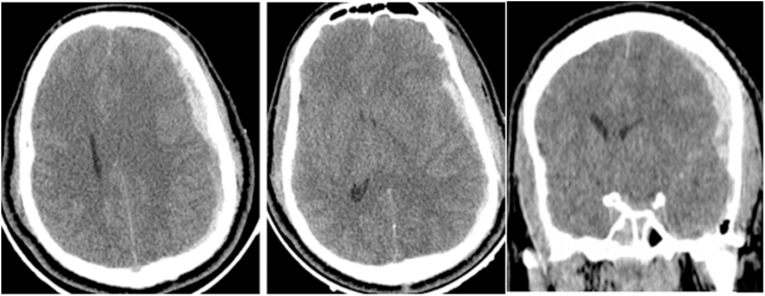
Admission CT Brain revealed left ASDH with midline shift associated to small Parieto-temporal EDH and linear fracture of the contralateral side.
